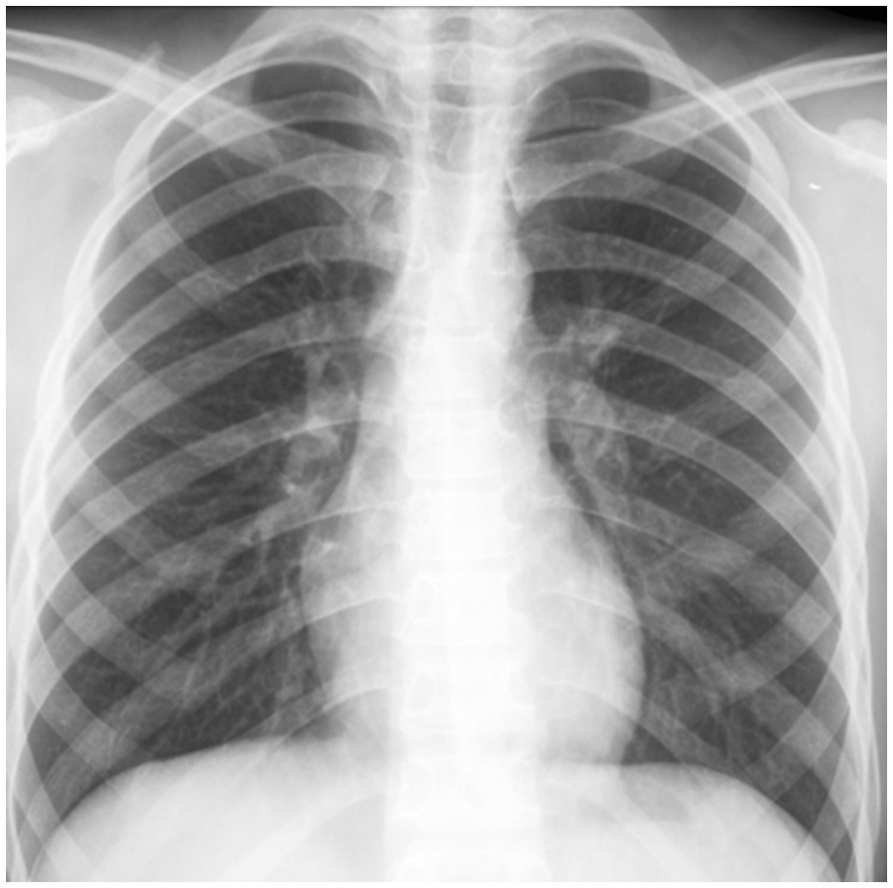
Chest radiograph without evidence of pulmonary involvement.
